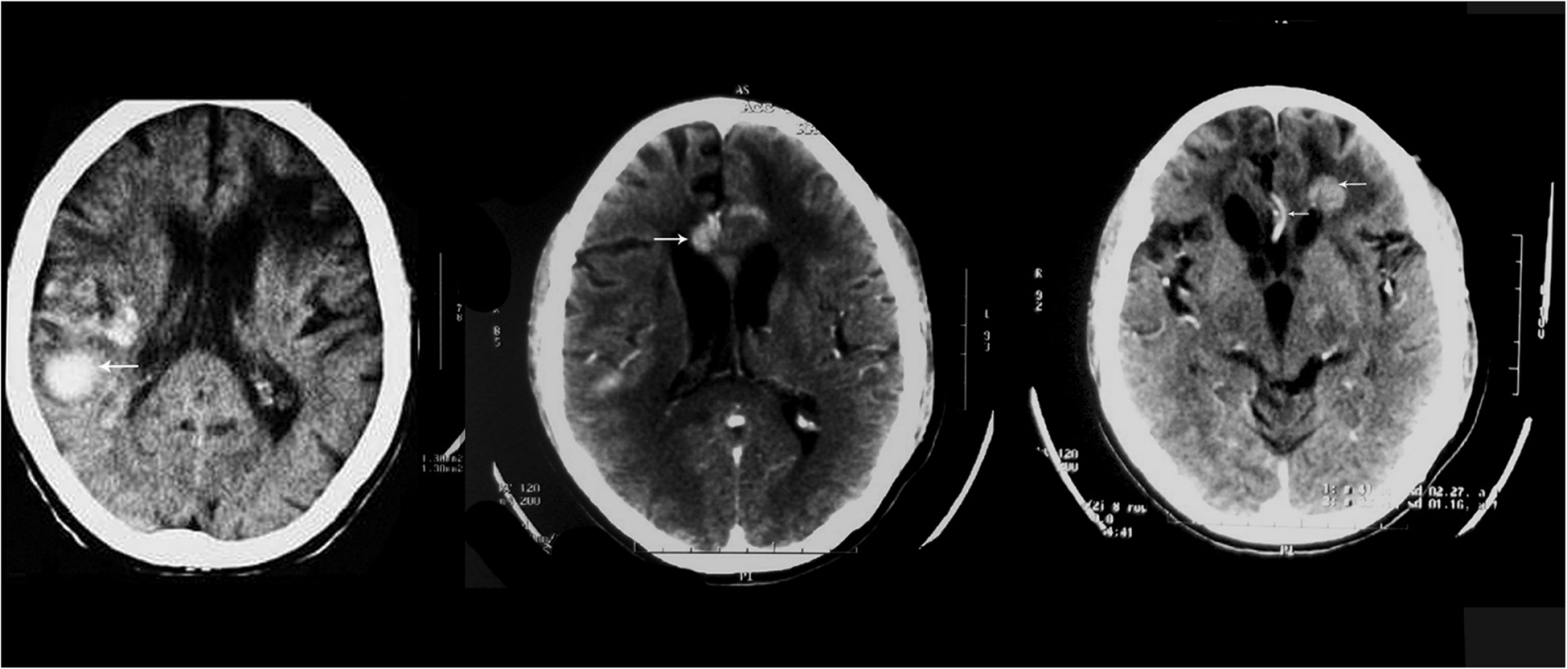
Head computed tomography scan showing multiple intraparenchymal hemorrhages (hyperdense areas) with surrounding hypodensities in the left frontal, right parietal and corpus callosum regions (arrows).
